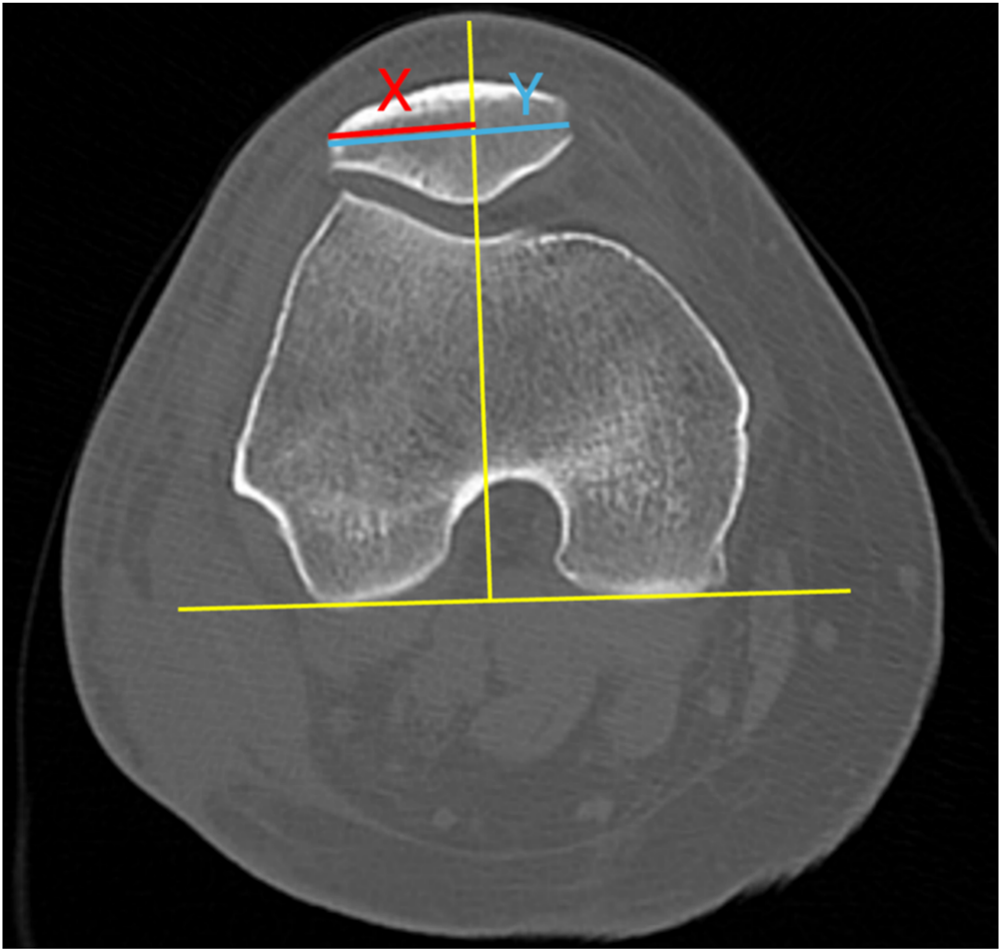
Measurement of the patellar bisect offset (BO) on an axial computed tomography image, adapted from Brossmann et al. [4]. A tangent to the posterior femoral condyles is drawn at the cut showing the perfect Roman arch. A line perpendicular to this tangent, intersecting the trochlea at its deepest point is drawn. Distances *X* and *Y* are then measured along the horizontal axis of the patella. BO (%) = *X/Y* × 100.
